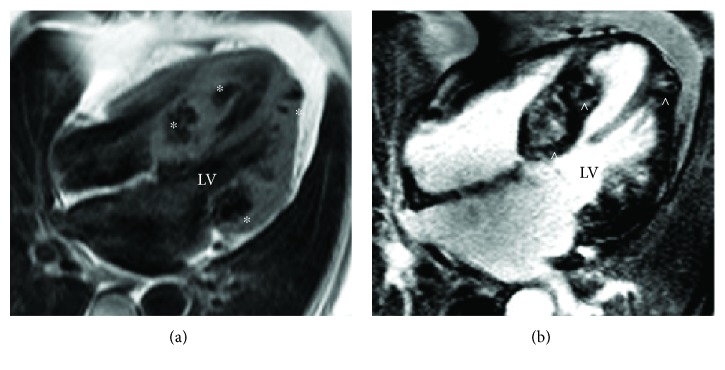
(a) Magnetic resonance T1-weighted spin echo demonstrating multiple low signal lesions throughout the left ventricular myocardium (^∗^), consistent with extensive infiltrative calcification of the myocardium. (b) Magnetic resonance phase-sensitive inversion recovery late gadolinium enhancement demonstrating a heterogenous composition to the intramyocardial lesions within the nulled myocardium (^), suggestive of caseating calcification within the myocardium (four-chamber view Siemens Avanto 1.5 Tesla).
